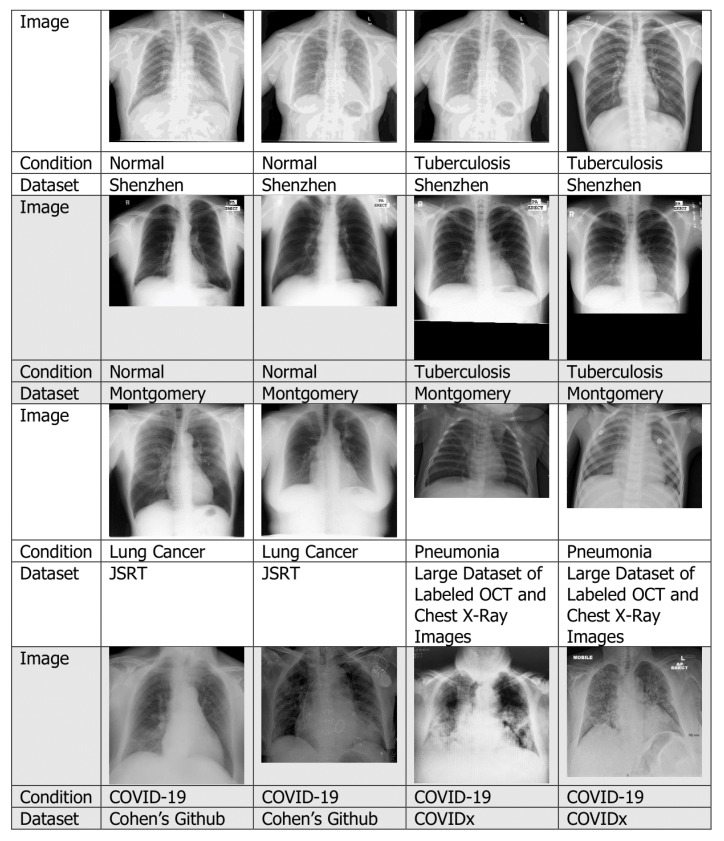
Examples of chest X-ray images.
